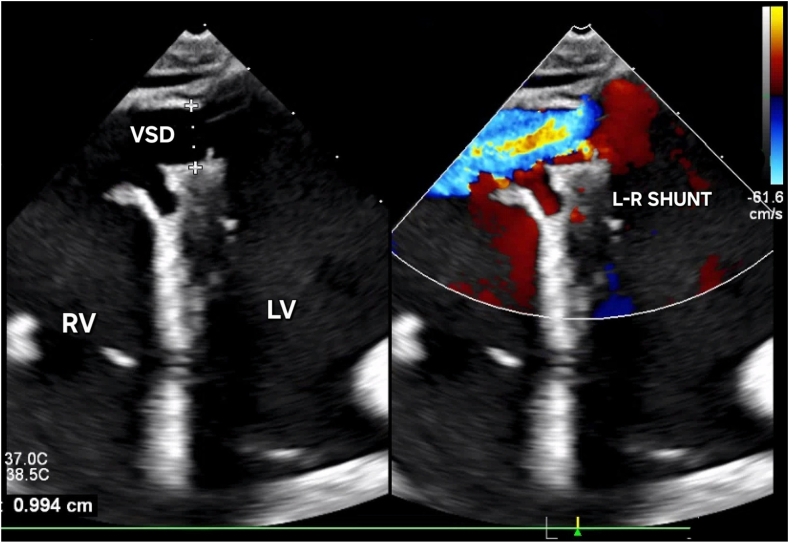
Transthoracic echocardiogram (on presentation) showing 1-cm VSD with left-to-right shunt. L-R shunt, left-to-right shunt; LV, left ventricle; RV, right ventricle; VSD, ventricular septal defect.
